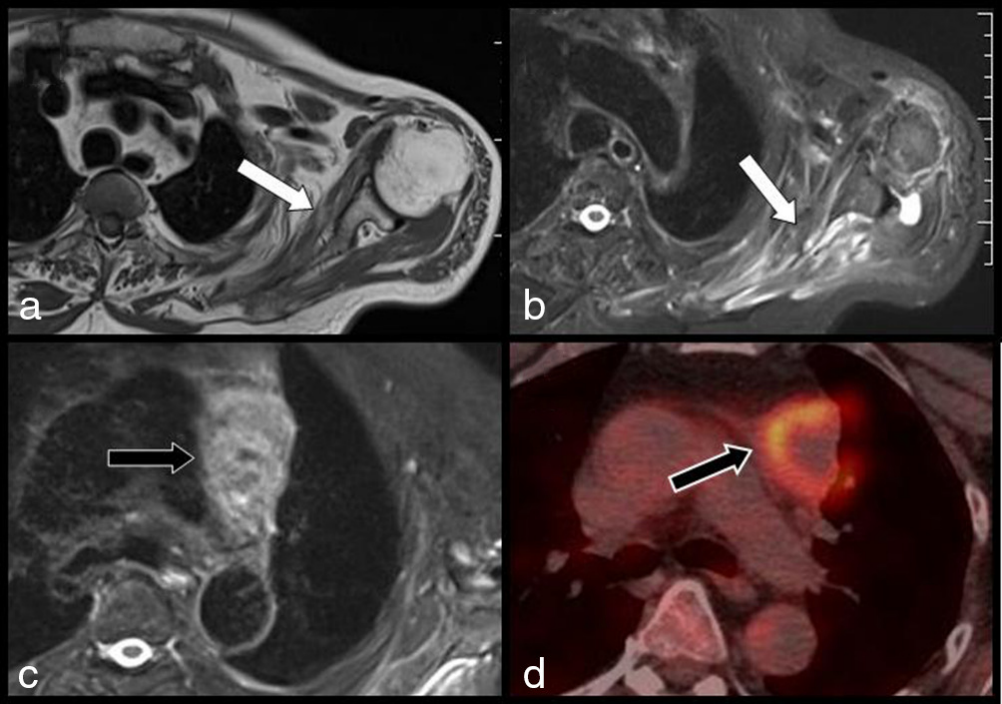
MRI views (a, b) of the left scapular area. *T*_1_ weighted image (a) showing diffuse atrophy of the scapular muscles (white arrow) and (b) typical denervation oedema (white arrow) is seen on *T*_2_ weighted image. *T*_2_ weighted image obtained more distally (c) in the mediastinum reveals a tumoral mass (black arrow) in the inferolateral portion of the anterior mediastinum. Corresponding 18-fludeoxyglucose–positron emission tomography/CT image (d) confirms the hypermetabolic tumoral mass (black arrow).
